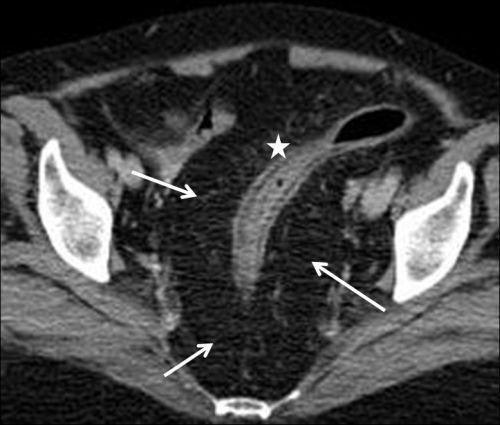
Pelvic computed tomography (CT) scan showing compression of the sigmoid colon (star) by an unencapsulated, homogenous soft tissue mass (white arrows).
